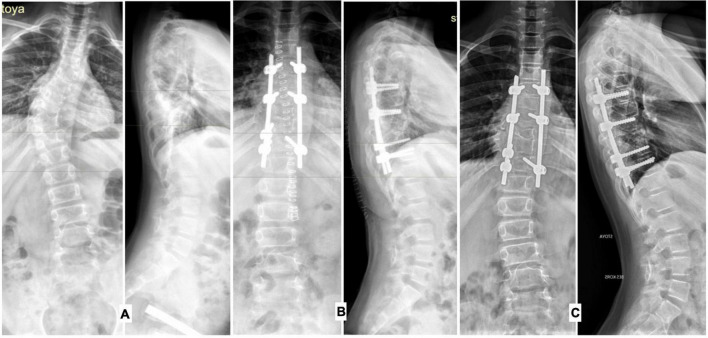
Radiographs of the spine in frontal and lateral projections, patient operated at the age of 9 years 6 months: (A) before surgical treatment, (B) on the 7th day after surgical treatment, (C) 1.5 years after correction of congenital deformity spine (dysplastic deformity of the spine below the zone of metal fixation).
